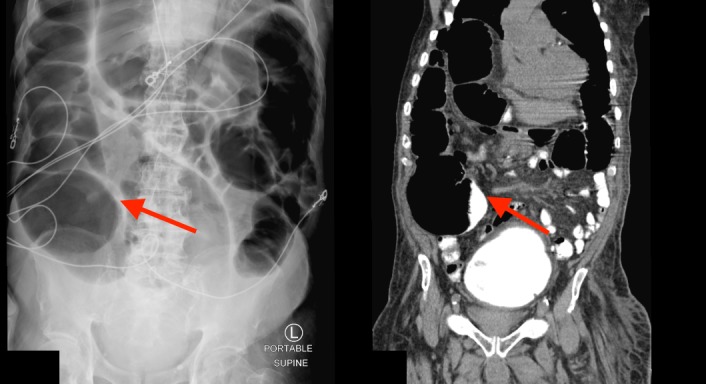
Abdominal radiograph and computed tomography scan (sagittal view) showing colonic pseudo-obstruction.
